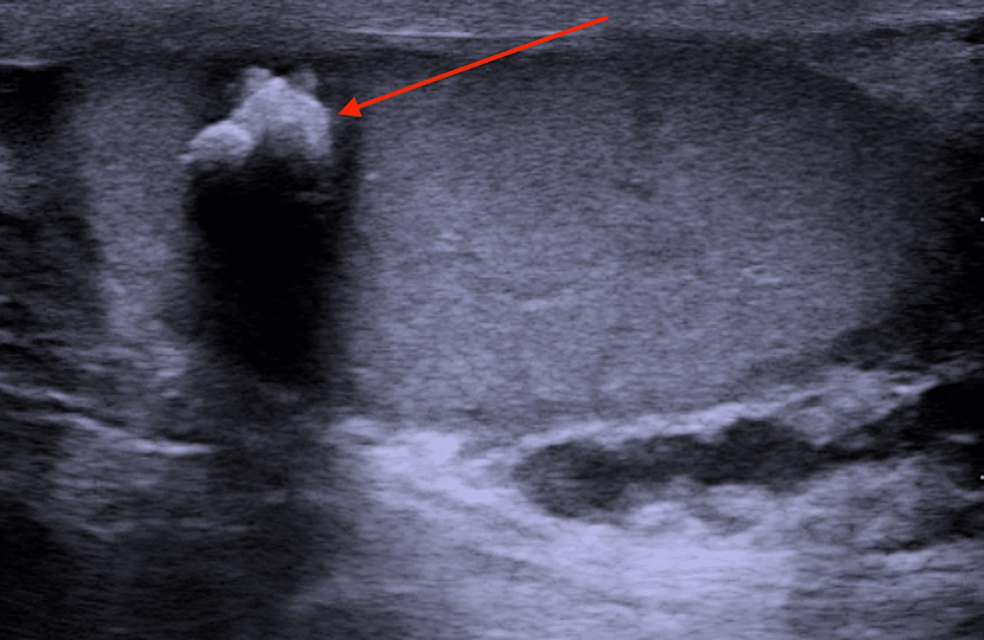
Ultrasound of the right testis Ultrasound image showing an 8 mm, right testicular calcified lesion (marked with the arrow)
